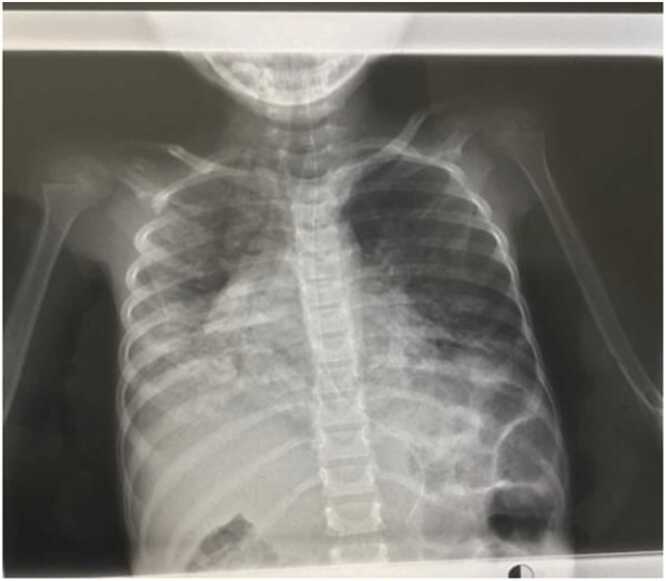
Chest radiograph showing pneumomediastinum with subcutaneous emphysema in a 30-month-old female patient with measles complicated by severe malaria (Case 6).
